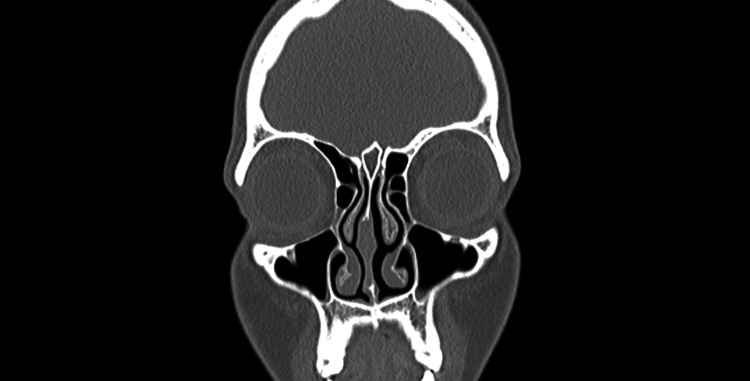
A CT scan of the sinuses did not show any abnormalities
